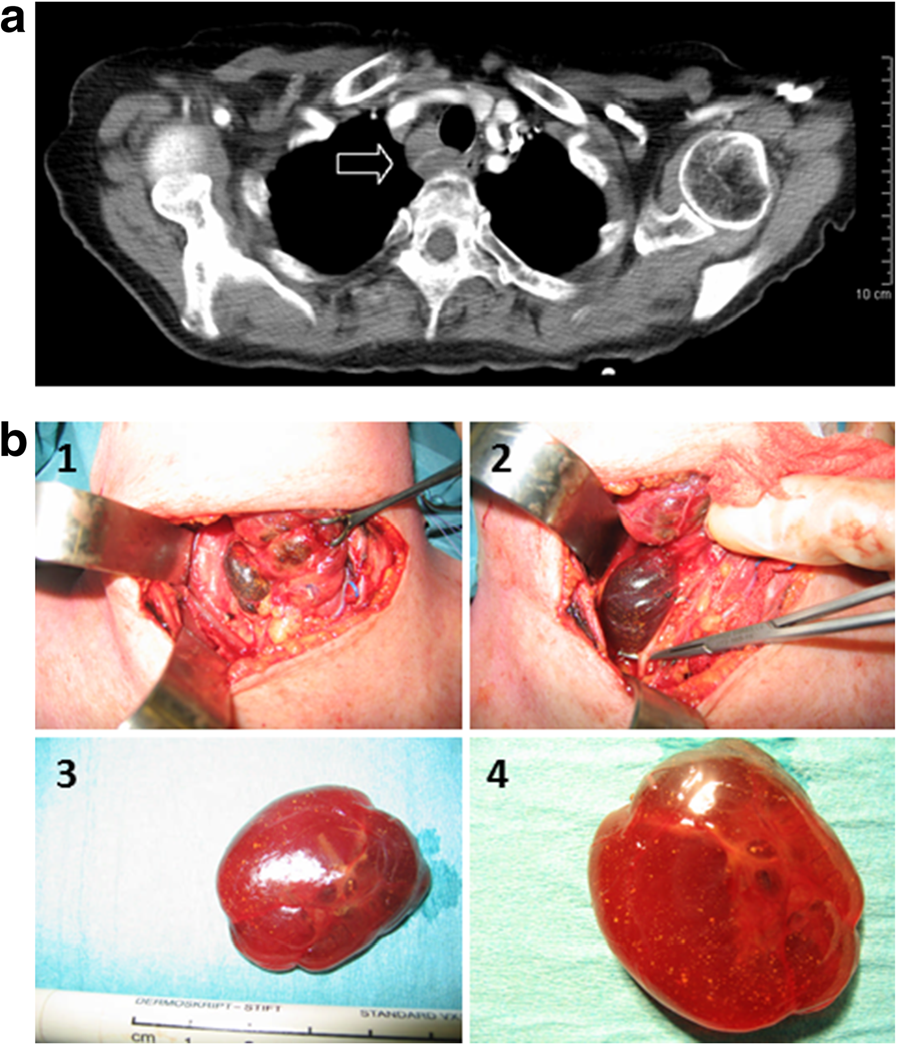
Patient 1. a Cervical computed tomography scan showing a paratracheal and paraesophageal cystic tumor with compression of the esophagus causing dysphagia (*arrow*). b Intraoperative view of the parathyroid cyst of patient 1 (panels 1 and 2). The cyst is located to the inferior thyroid artery and the laryngeal nerve. Right thyroid lobe is retracted to the left. Panels 3 and 4 Resected cyst with fine lining of parathyroid tissue
